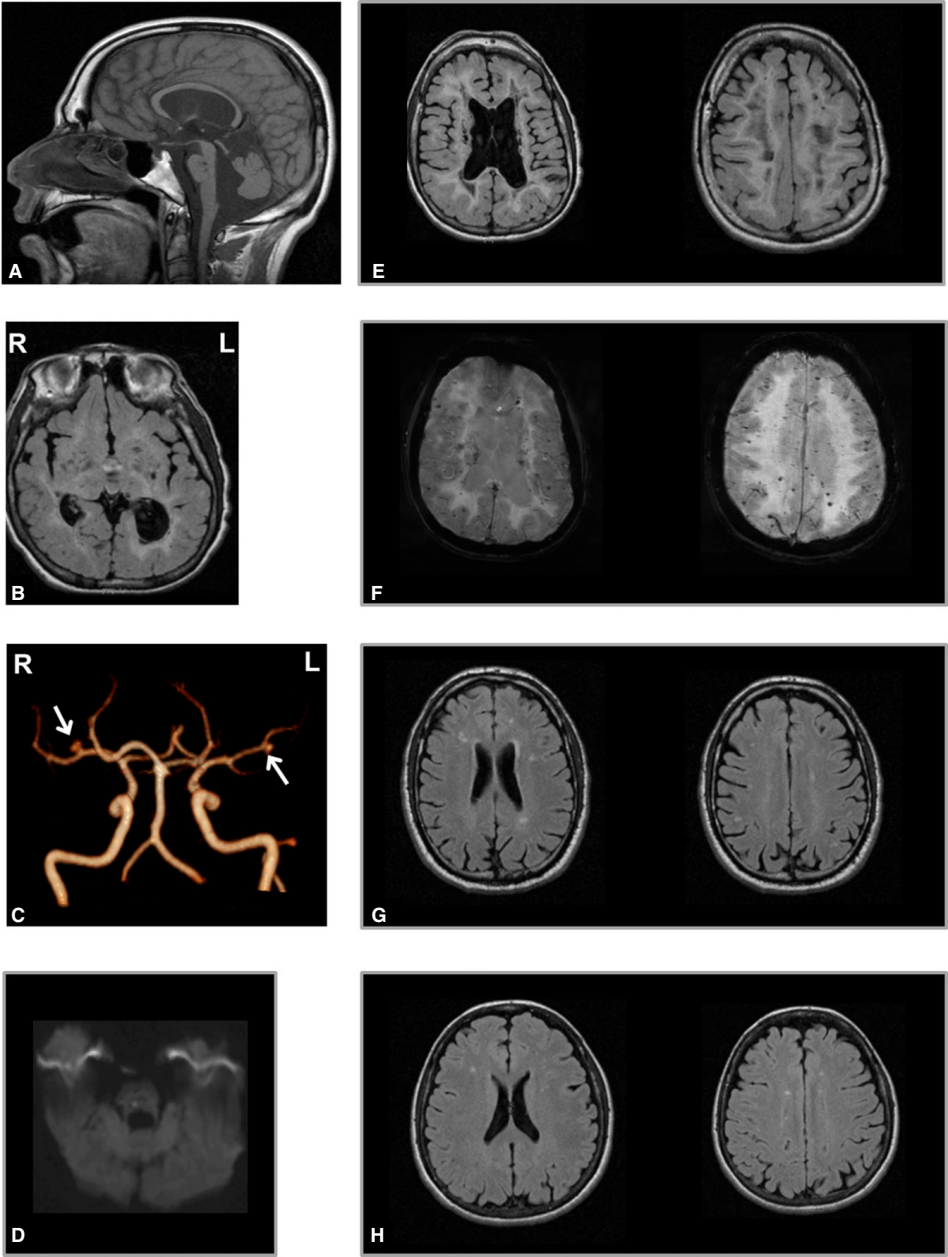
Brain MRI study of the proband and parents
